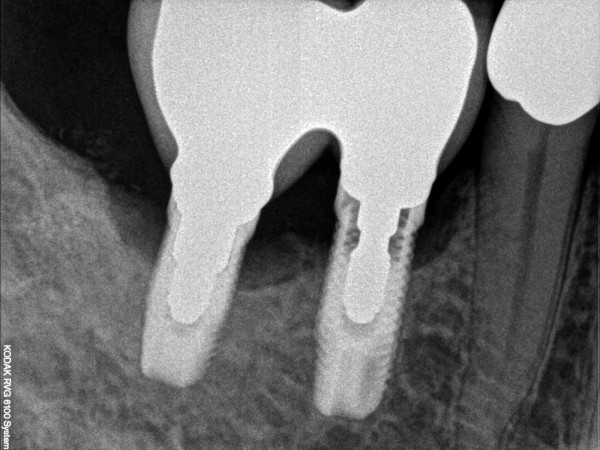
Preoperative radiograph of the peri-implant defect.
